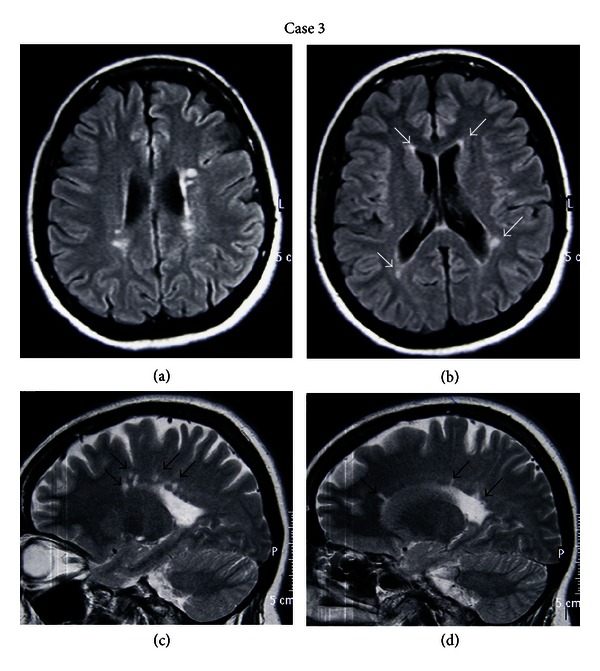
(a) and (b) Axial FLAIR brain MRI images of the third patient demonstrating multiple periventricular and subcortical lesions at the parietal and temporal lobes (white arrows). (c) and (d) Sagittal T2-weighted brain MRI images showing multiple lesions of the corpus callosum (black arrows).
